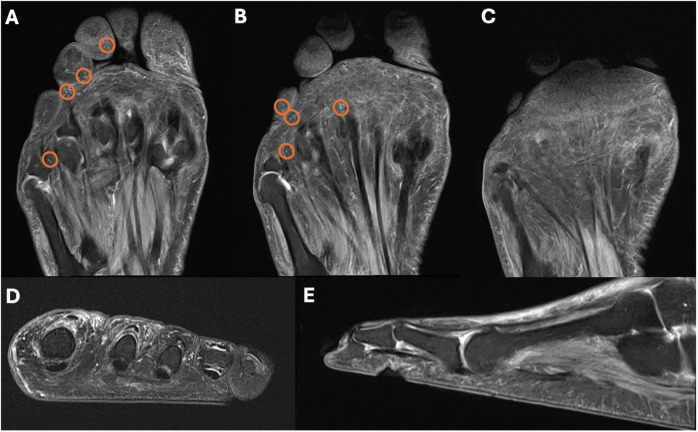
Severe rarefaction and disturbed arrangement of Pacinian corpuscles (PC) on forefoot MRI of a patient with diabetic sensorimotor polyneuropathy (DSP). Consecutive coronal proton-density (PD)-weighted fat-saturated (fs) images (A–C), axial T2-weighted fs image (at the level of the proximal phalanges) (D), and sagittal PD-weighted fs (at the level of the third digit) (E) image of the left foot of a 66-year-old male with DSP, who was diagnosed with type 2 diabetes 8 years ago. MRI demonstrates a severe reduction of PC numbers in the subcutaneous fat and along the deep muscle fascia, as well as an altered PC distribution (“spot-like” pattern): Only a few randomly distributed PC are visible in the plantar subcutaneous fat (circles A, B). As of note, these all had a small diameter (1–2 mm)
